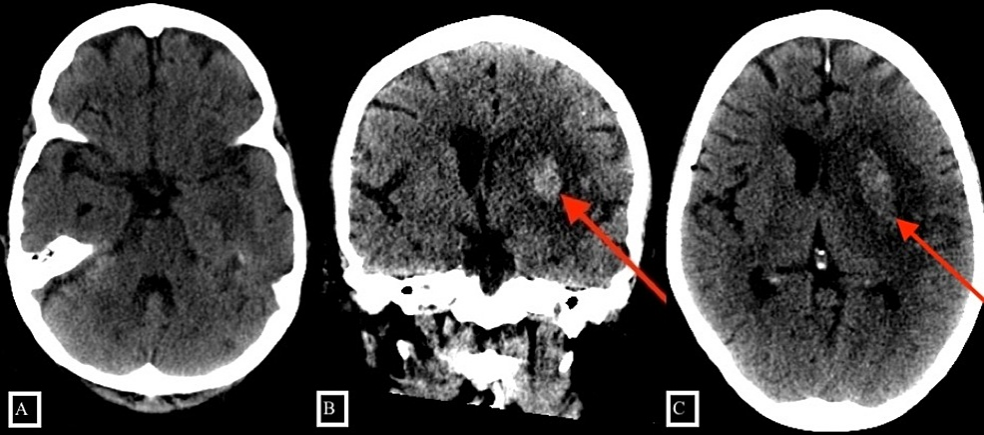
A 24-hour post tPA CT of the head shows no evidence of calcified emboli in the left M1 segment with intraparenchymal hemorrhage in the left putamen. tPA, tissue plasminogen activator
